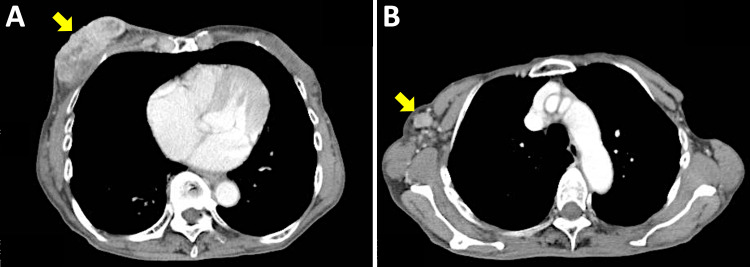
Contrast-enhanced computed tomography image obtained at the time of referral to the radiation oncology unit. (A) The primary tumor in the right breast (70 x 24 mm). (B) Metastasis to a right axial lymph node (15 x 11 mm). Arrows indicate the tumors.
